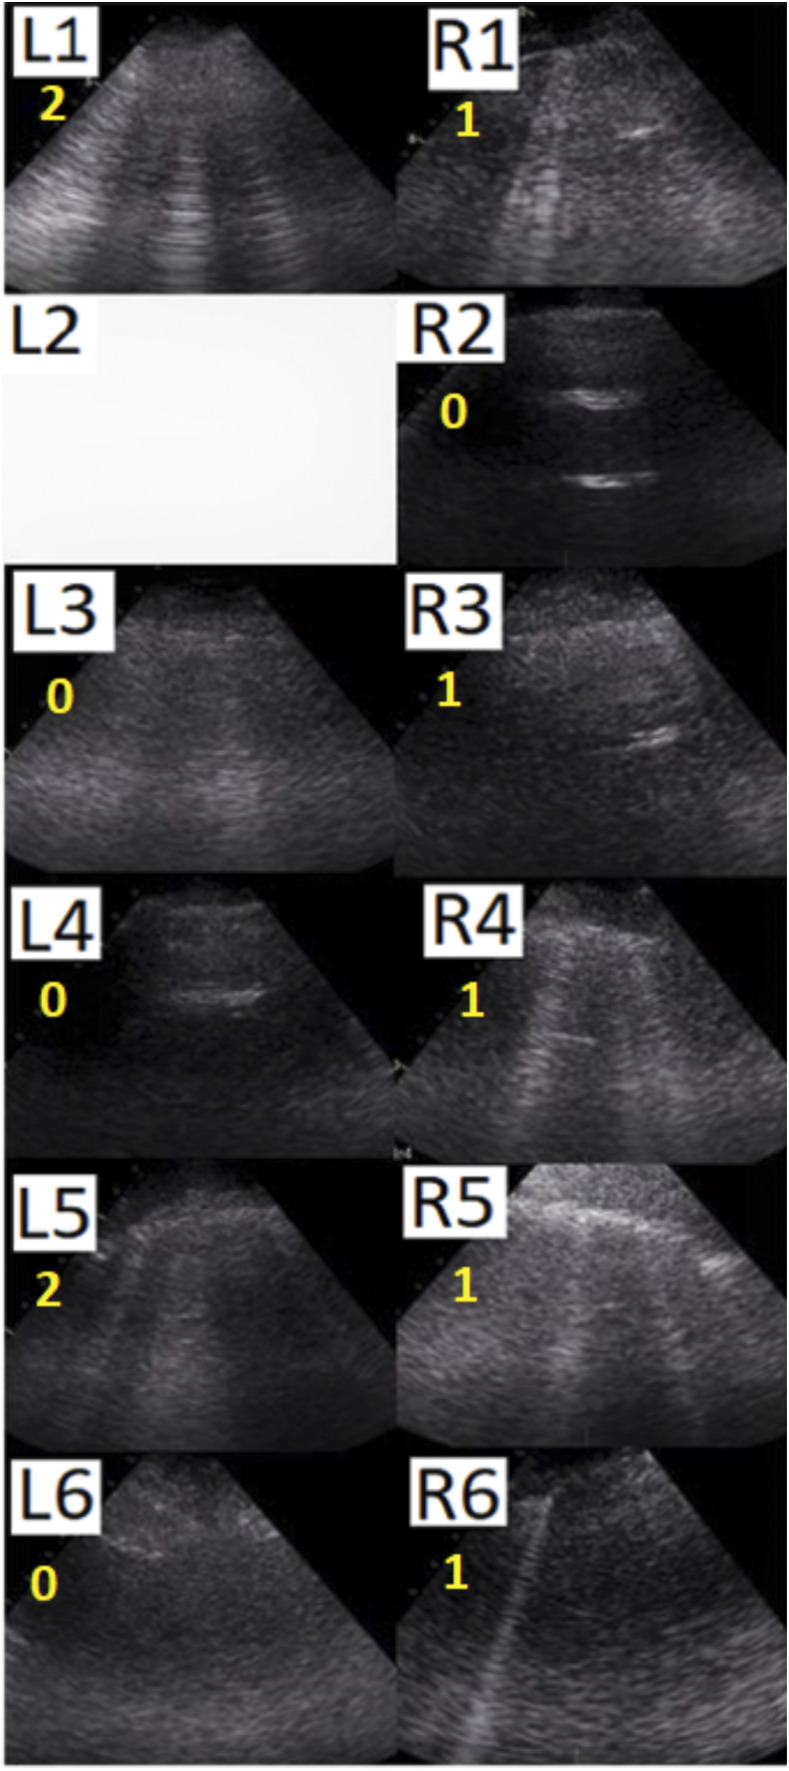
Lung ultrasound images for patient B. L = left hemithorax; R = right hemithorax; 1, 2 = anterior thoracic areas; 3, 4 = lateral thoracic areas; 5, 6 = posterior thoracic areas.
